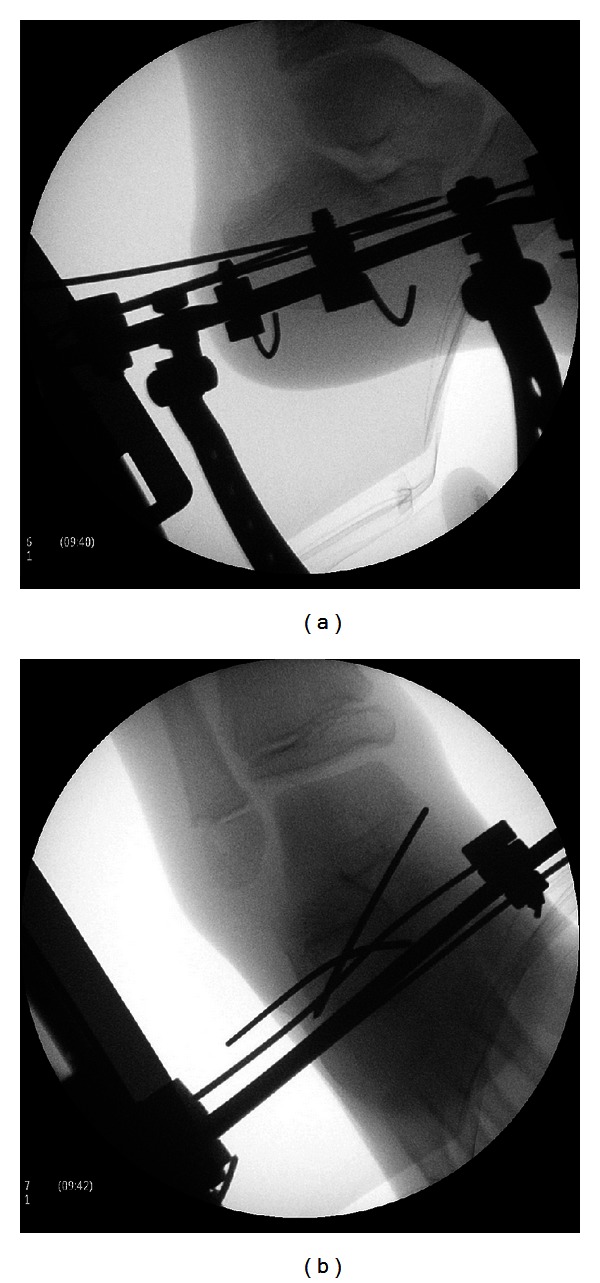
(a) Lateral radiograph confirming anatomical reduction. (b) Anteroposterior radiograph confirming anatomical reduction and demonstrating position of K-wires.
